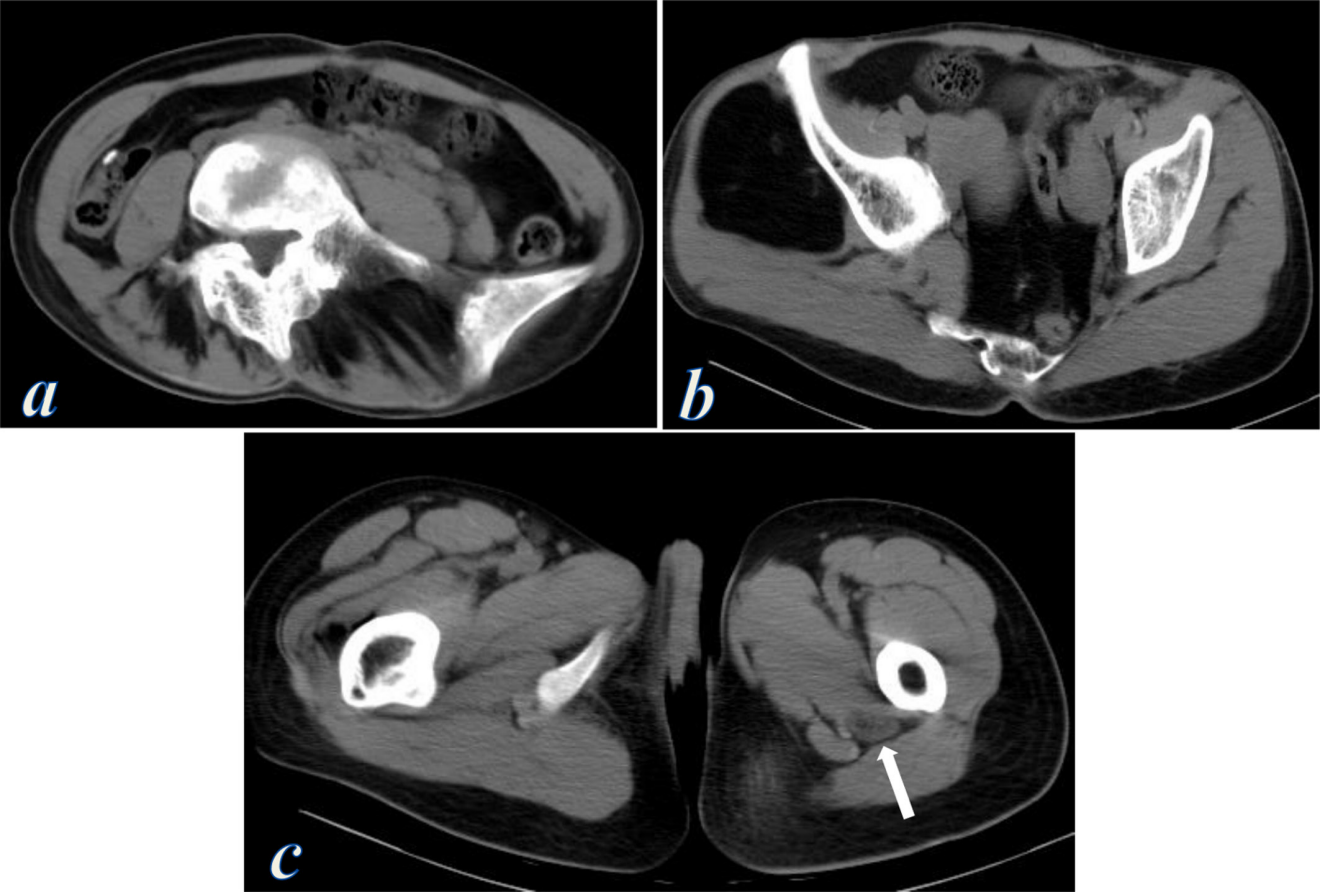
CT scan of the abdomen, pelvis, and thighs. (a) Axial image of the abdomen shows fatty infiltration of the erector spinae muscles. (b) Axial images of the pelvis show asymmetric intra-abdominal and pelvic lipomatosis (evident on the right paracolic gutter and pararectal regions) and a large right gluteal intramuscular lipoma. (c) Axial image of both thighs shows diffuse enlargement of the left sciatic nerve showing fatty infiltration (arrow).
